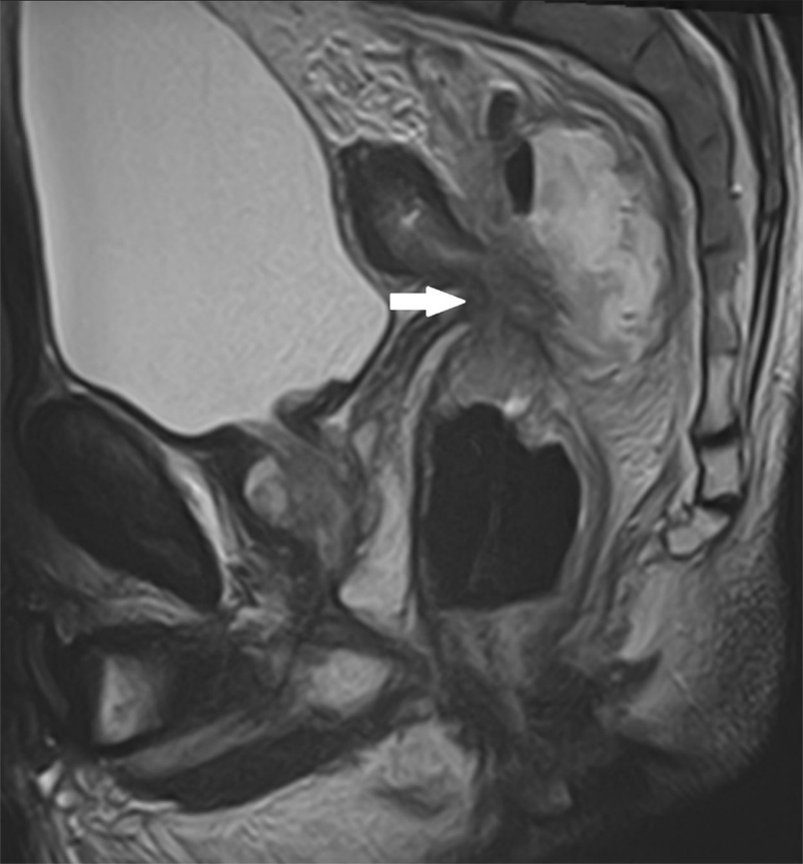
Sagittal *T*_2_ weighted MRI: arrow shows rectal tumour with an abscess posterior to the tumour.
